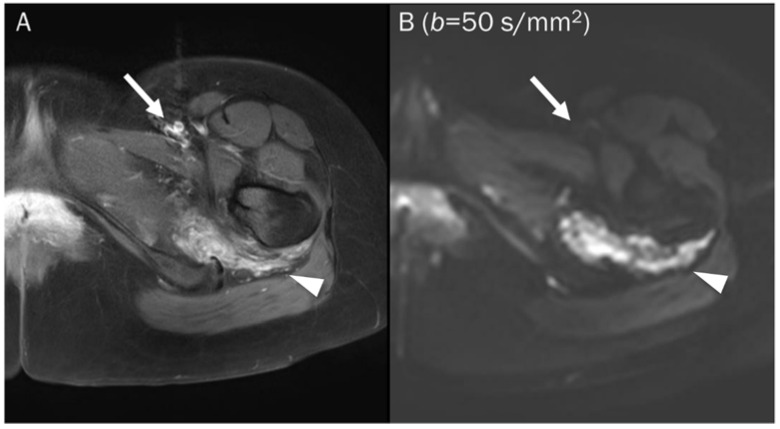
A 25-year-old female with a soft tissue hemangioma in the proximal thigh. (A) Axial T1-weighted enhanced image shows hemangioma (arrowhead) at the quadratus femoris muscle and femoral artery and vein (arrow) at the inguinal area. (B) DWI with a *b*-value of 50 s/mm^2^ image still exhibits a signal of the hemangioma (arrowhead) due to slow blood flow within the vessels, while the femoral artery and vein (arrow) are invisible due to fast-flowing blood within the vessels, creating a “black blood” effect.
